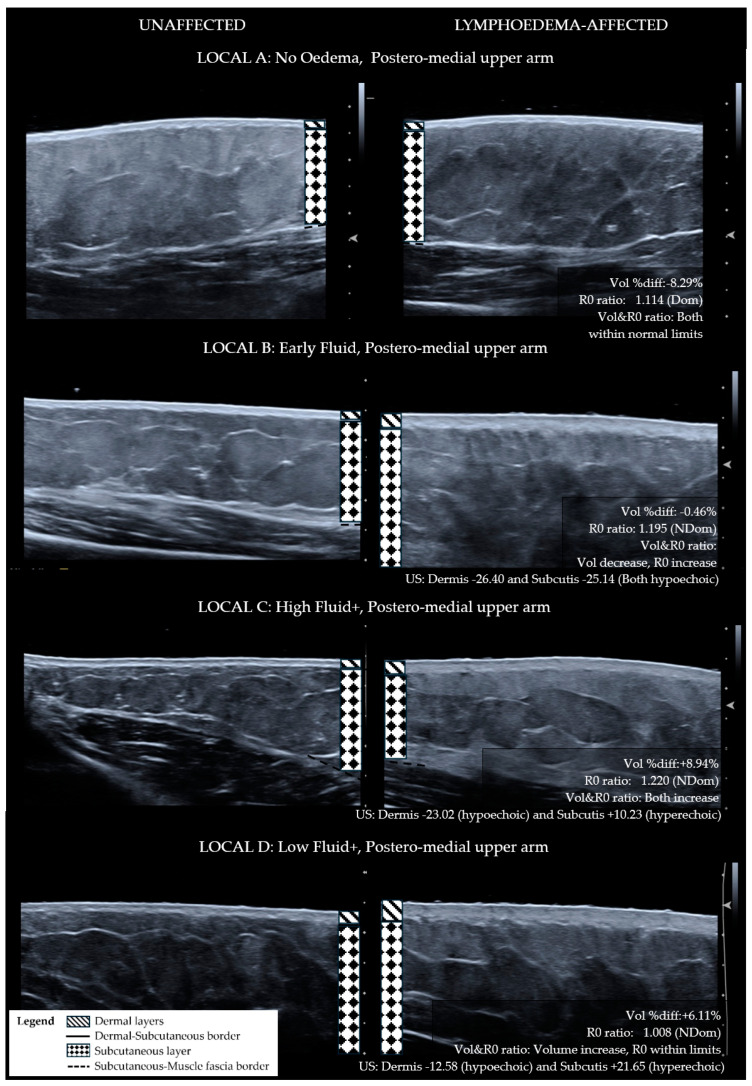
Ultrasound images for postero-medial upper arm sites categorised with LOCAL criteria—matched lymphoedema-affected and unaffected sites. Lymphoedema-unaffected site (left), lymphoedema-affected test site (right). LOCAL A: No tissue characteristic change. LOCAL B: Lymphoedema dermal thickness increase and full-thickness hypo-echogenicity of the subcutis compared to unaffected site. Serrated appearance of the dermal–subcutaneous border. LOCAL C: Lymphoedema dermal thickness increase with hypoechogenic changes to both tissue layers. Discord in echogenicity within the subcutis. LOCAL D: Lymphoedema dermal thickness increase with hyper-echoic serrated appearance of the dermal–subcutaneous border.
